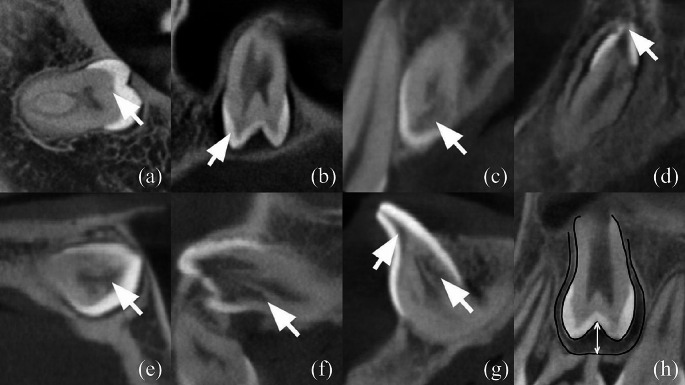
PEIR classification from CBCT images. (a) PEIR Score I, (b) PEIR Score II, (c) PEIR Score III, (d) PEIR Score IV, (e) PEIR Score V, (f) Root Extension, (g) 2 Lesions in 1 Affected Teeth, (h) Distance for Pericoronal Space Measurement
